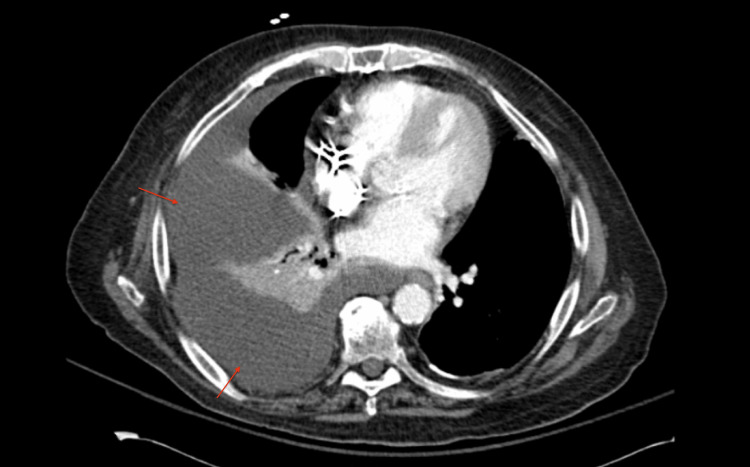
Axial CT with contrast showing large right pleural effusion and adjacent atelectasis.
